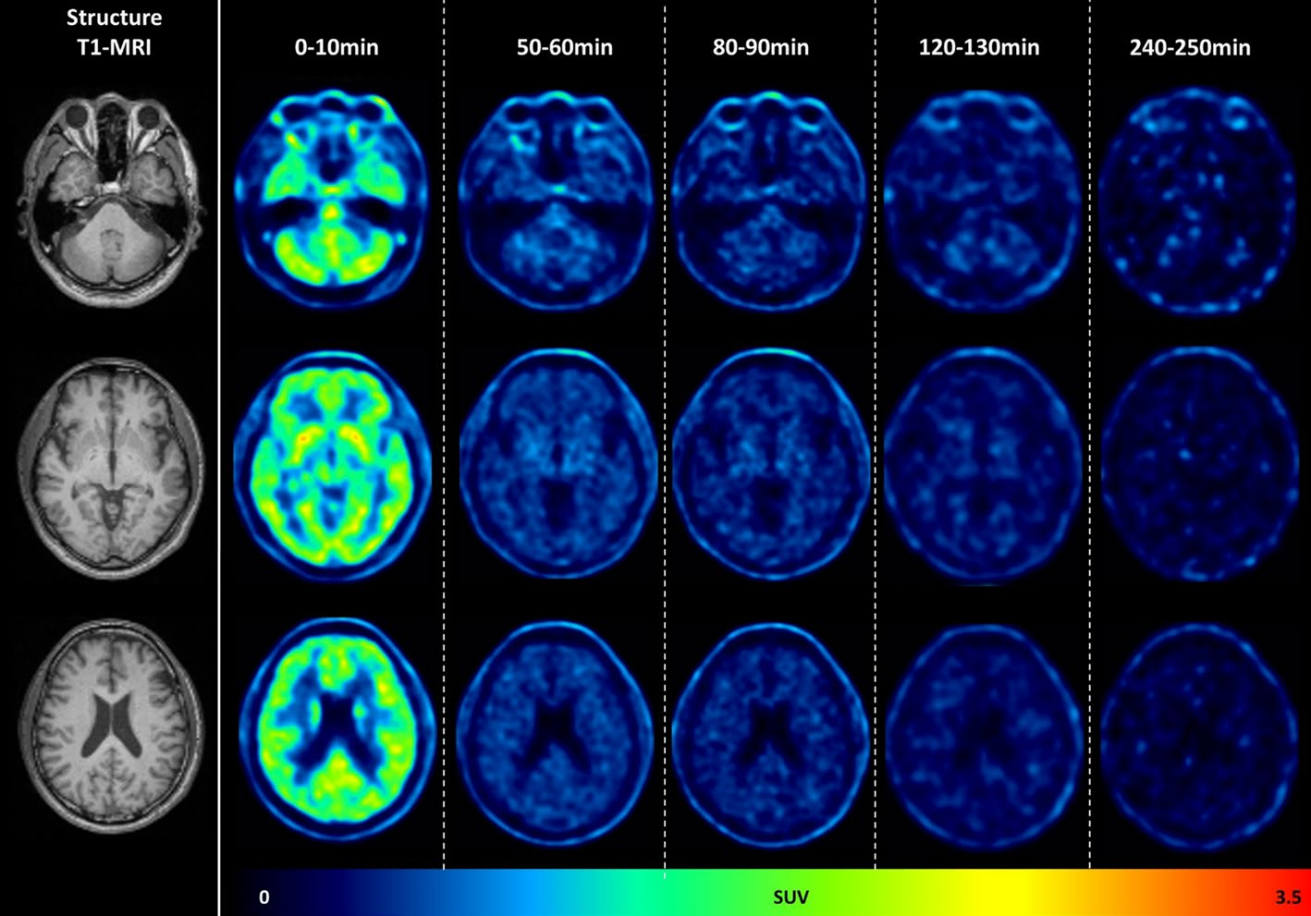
A T1-weighting MRI and dynamic brain PET images of a 65-year-old man. Initially, At the outset, the brain exhibited the highest tracer uptake within the first 10 min post-injection, followed by a gradual decrease in uptake over time, and at 240 min post-injection, there was significant tracer clearance. The left images are T1-MR images in different slices; the right images are corresponding dynamic brain PET obtained at the time intervals of 0–10 min, 50–60 min, 80–90 min, 120–130 min, and 240–250 min post-injection of [^18^F]Florzolotau. All PET images are displayed in the SUV scale
